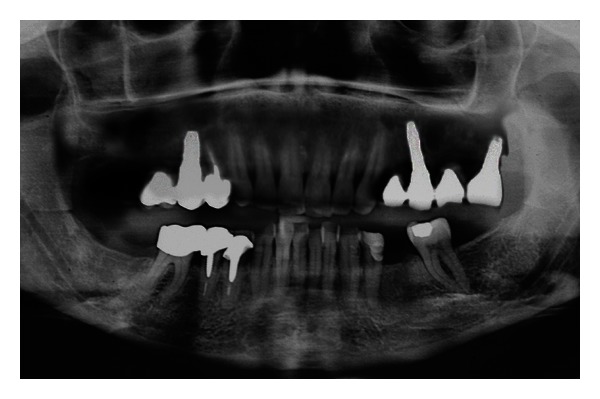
Panoramic X-ray after the intervention.
